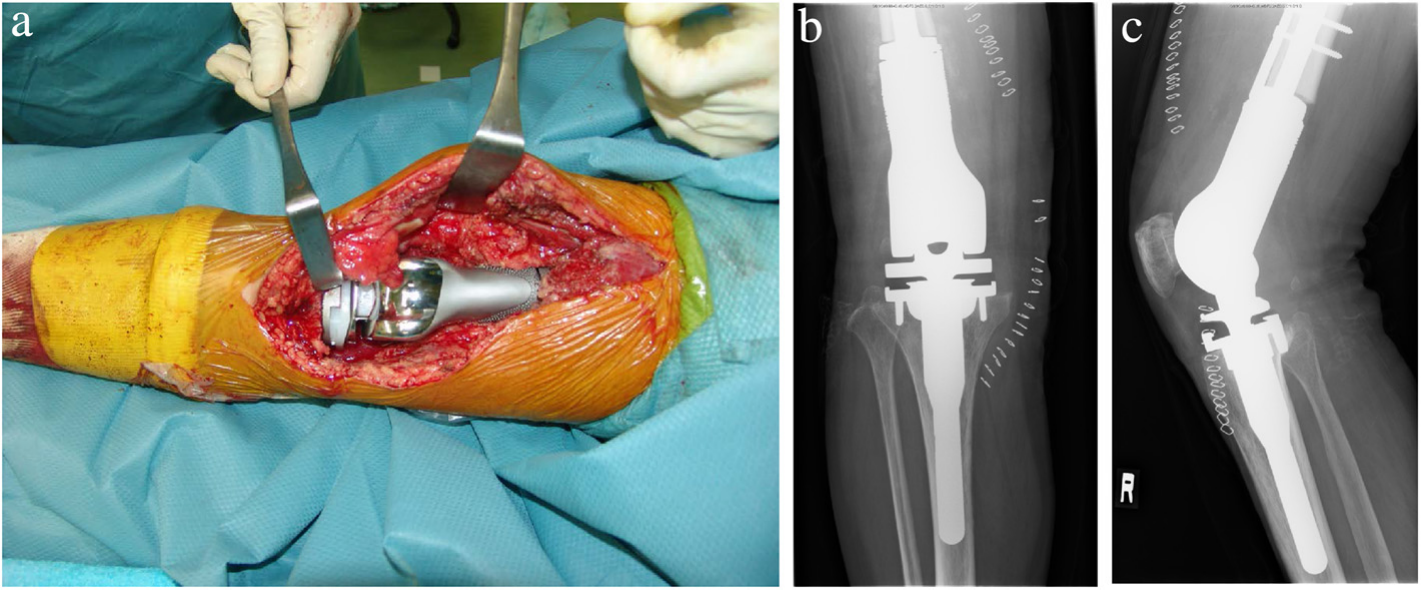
Intraoperative finding. Wide resection of the tumor was performed and was reconstructed with a tumor endoprosthesis (a). Anteroposterior (b) and lateral (c) radiograph after operation
